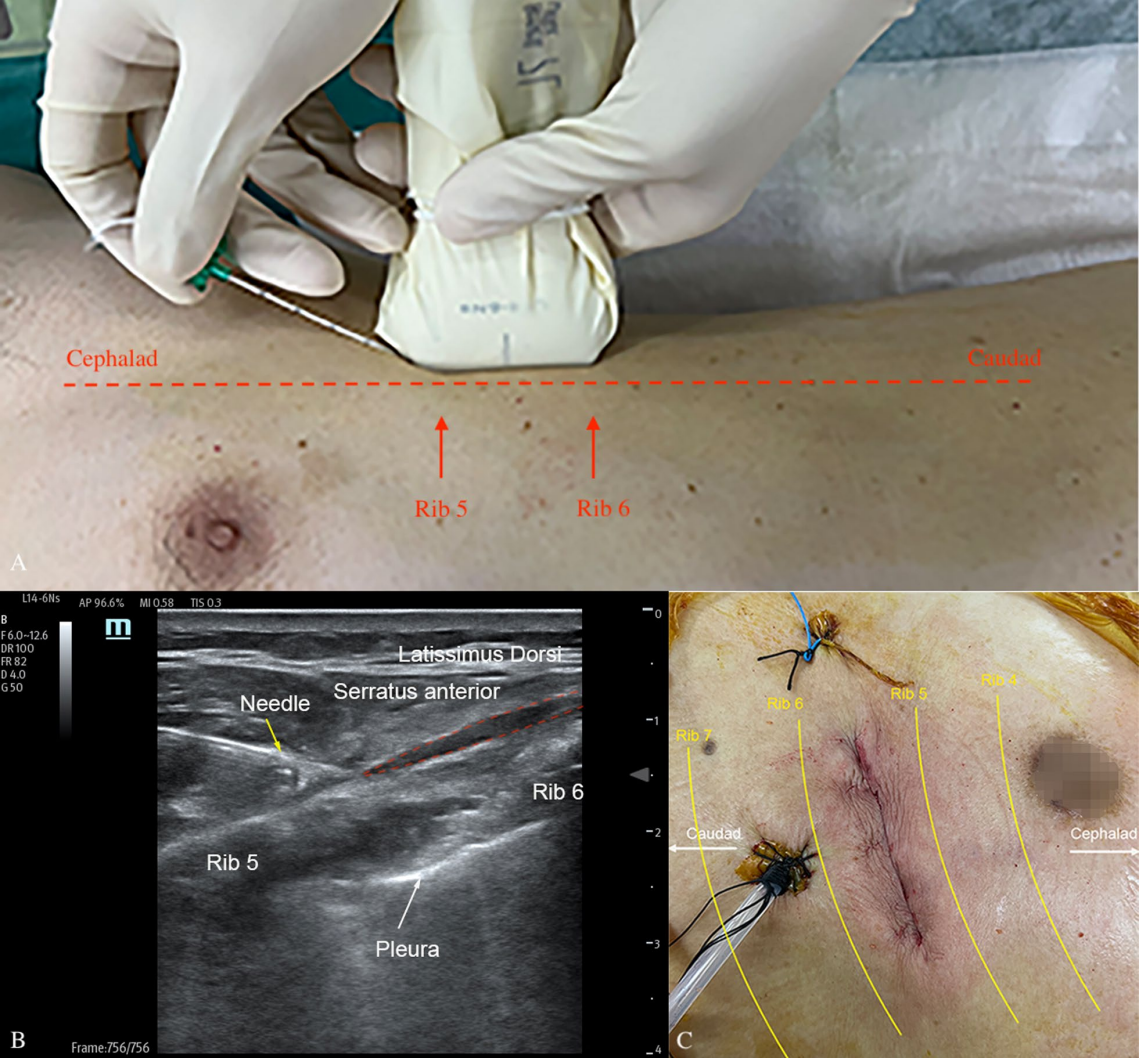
Serratus anterior plane block. A Location of administration of the serratus anterior plane block. B Ultrasound image of the serratus anterior plane block. The yellow arrow indicates the needle. The area of red dotted line indicates the spread of local anesthetics. C The left mini-thoracotomy into left ventricular apex
